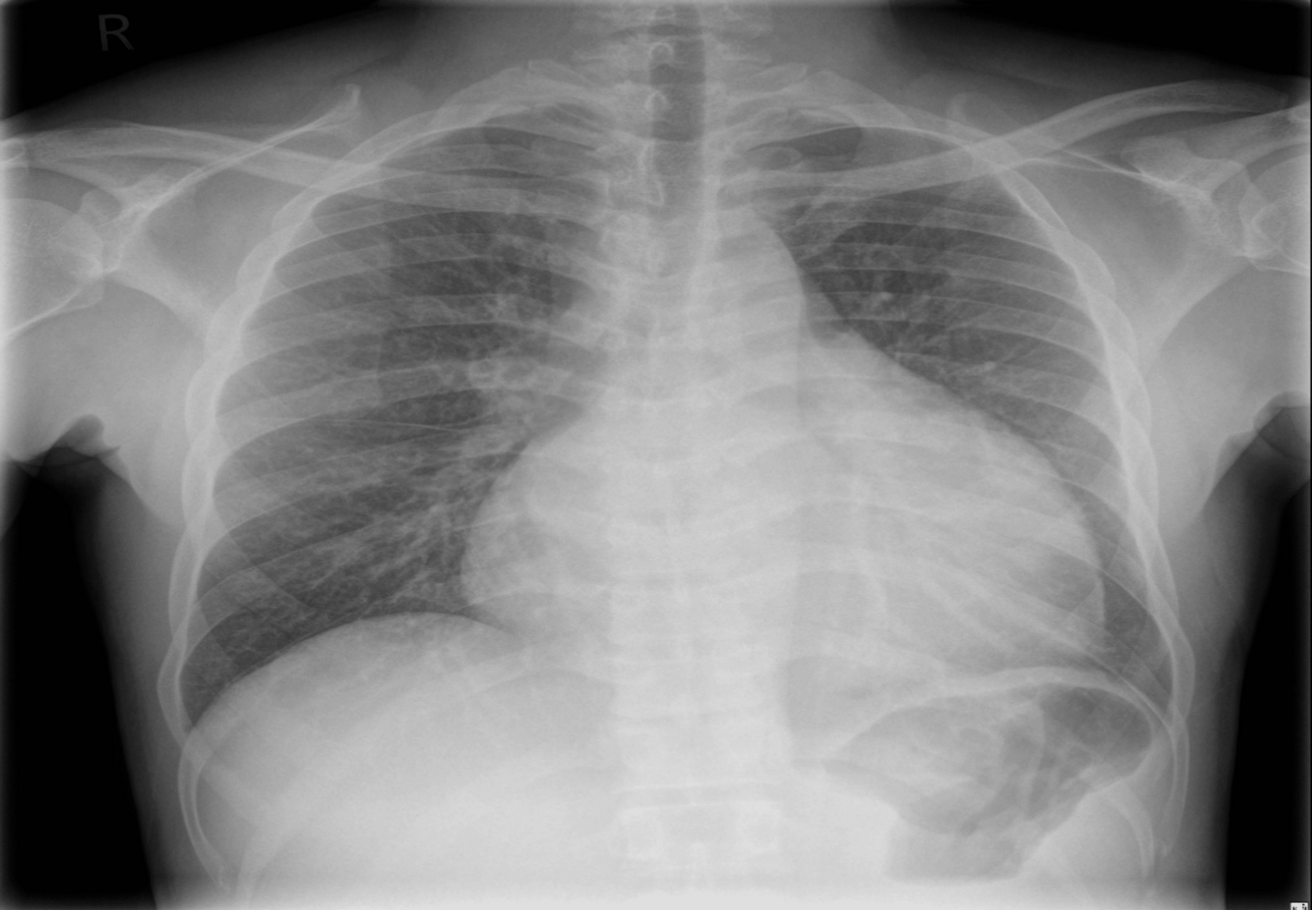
Chest X-Ray Frontal chest radiograph demonstrates an enlarged cardiac silhouette, suggestive of pericardial effusion. A small amount of fluid is visible in the horizontal fissure. No pulmonary infiltrates or cavitary lesions are identified.
